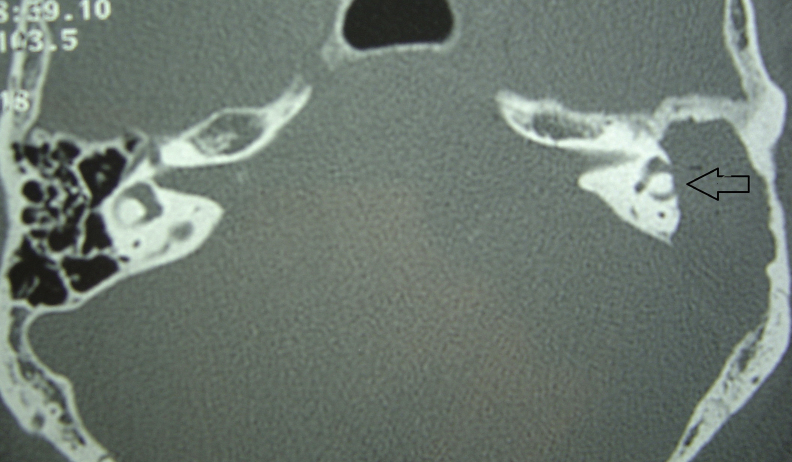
Temporal bones CT, axial view. The arrow shows erosion of the lateral semicircular canal on the left, in a patient with CCOM. *Note*: posterior erosion in the mastoid, adjacent to the sigmoid sinus.
